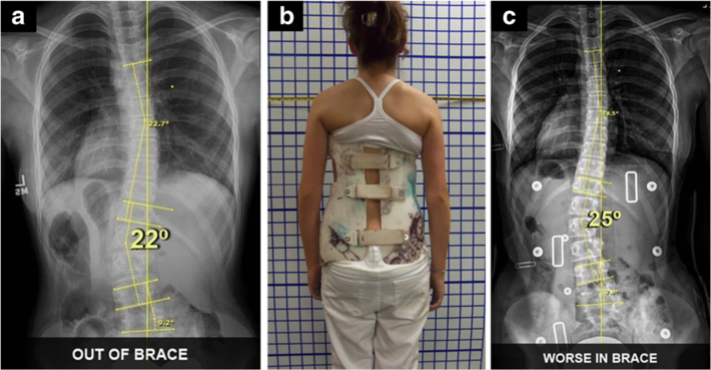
TLSO-type brace with low axilla, thoracic and lumbar pads which presents a worse in-brace situation. a: Patient out-of-brace on July 2013 presented with a right thoracic curve of 22-degree Cobb angle at T7 to T12 with the curve apex at T9; and a left lumbar curve of 22-degree Cobb angle at T12 to L4 with the curve apex at L2-3. Although the upper end-plate maximum inclination was T7, the curve inclined up to T7-T6-T5. b: Patient on July 2013 in TLSO-type brace which the patient reported to be a comfortably fitting brace. c: The TLSO-type in-brace X-ray presented the thoracic pad at T10 with the pad pressures and size of pad covering approximately T10 to T12/L1, and the counterforce at the left axilla at T 7-8. As a result, the in-brace X-ray showed a scoliosis which was worse in-brace compared to out-of-brace. TLSO-type in-brace was thoracic 18.5 degree Cobb and lumbar 25 degree Cobb angles. This was the direct result of firstly, the axilla extension being too low, which caused the buckling effect, and secondly, the low thoracic pad blocking the lumbar correction. This configuration was causing lumbar curve progression in the brace.
